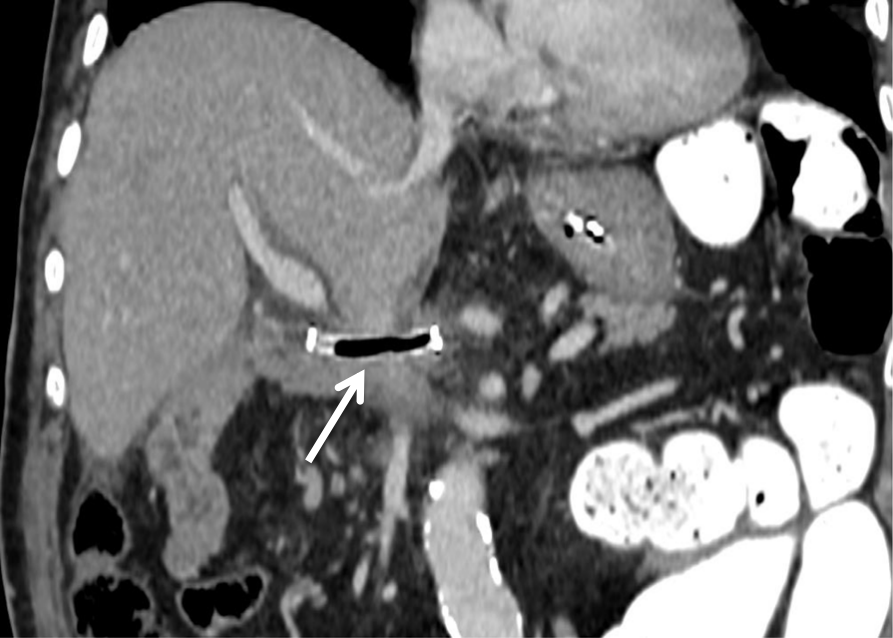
Enhanced CT on POD 85. CT reveals the presence of air within the occluded stent graft, which indicates stent graft infection (arrow)
